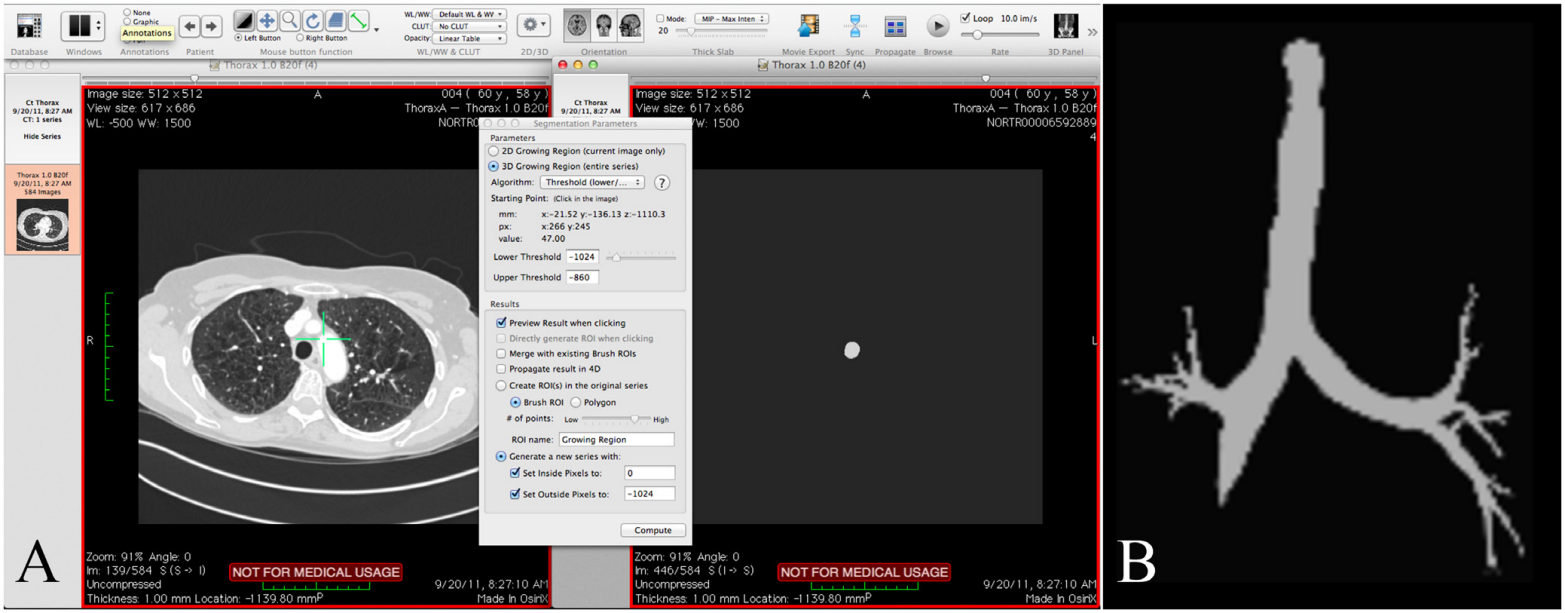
OsiriX GUI. A) axial view of the original CT data, 2D result of the segmentation with threshold upper/lower algorithm, window of the region growing with threshold (upper/lower) algorithm; B) 3D panel.
